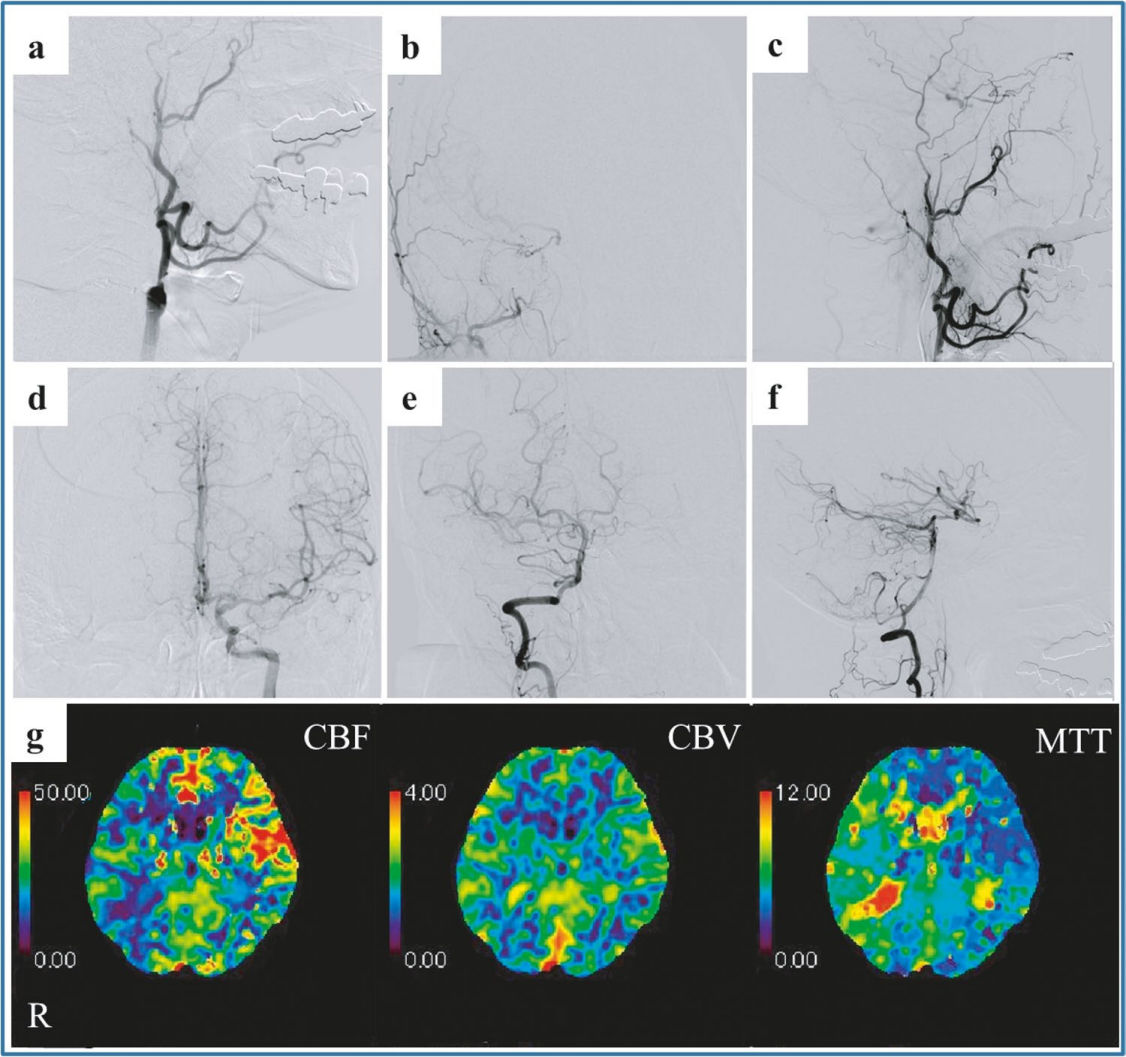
Angiography and perfusion computed tomography before treatment. (a–c) The right common carotid angiography revealed complete right ICA occlusion and formation of collateral circulation through reversed flow in the right ophthalmic artery from the right external carotid artery. (d) The left internal carotid angiography revealed closure of the right A1. (e–f) The right vertebral angiography revealed the right posterior communicating artery from the posterior circulation. (g) Perfusion computed tomography revealed the right cerebral hypoperfusion compared to the left
